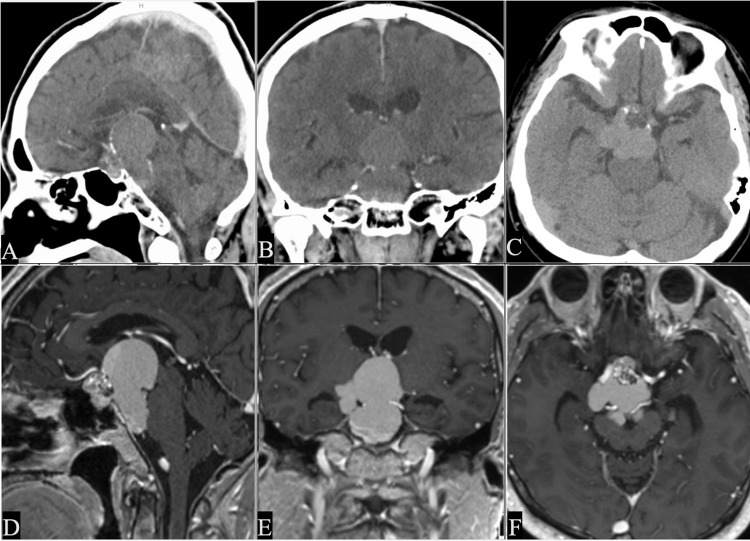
Preoperative CT and MRI (A-C) Preoperative CT and MRI imaging demonstrates the cystic lesion with calcifications suprasellar and retroinfundibular region. (D-F) Gadolinium-enhanced MRI shows a slightly heterogeneously enhanced area within the lesion, which was compressing the optic chiasm, third ventricle, and brainstem
